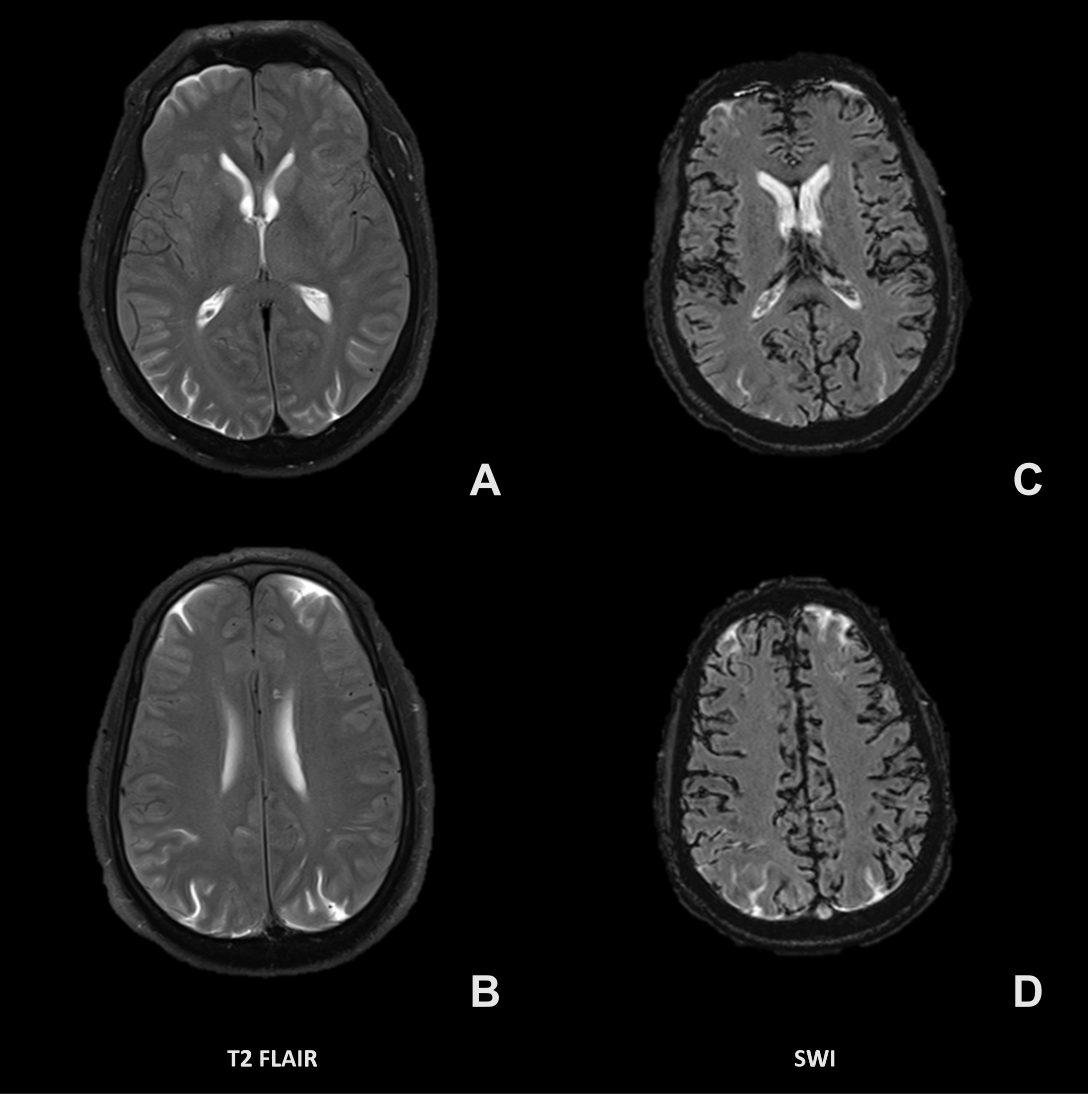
Non-contrast 1.5 T magnetic resonance imaging (MRI), taken 14 h after intrathecal blood injection, with both fluid-attenuated inversion recovery (FLAIR; a, b) and susceptibility weighted imaging (SWI; c, d)
